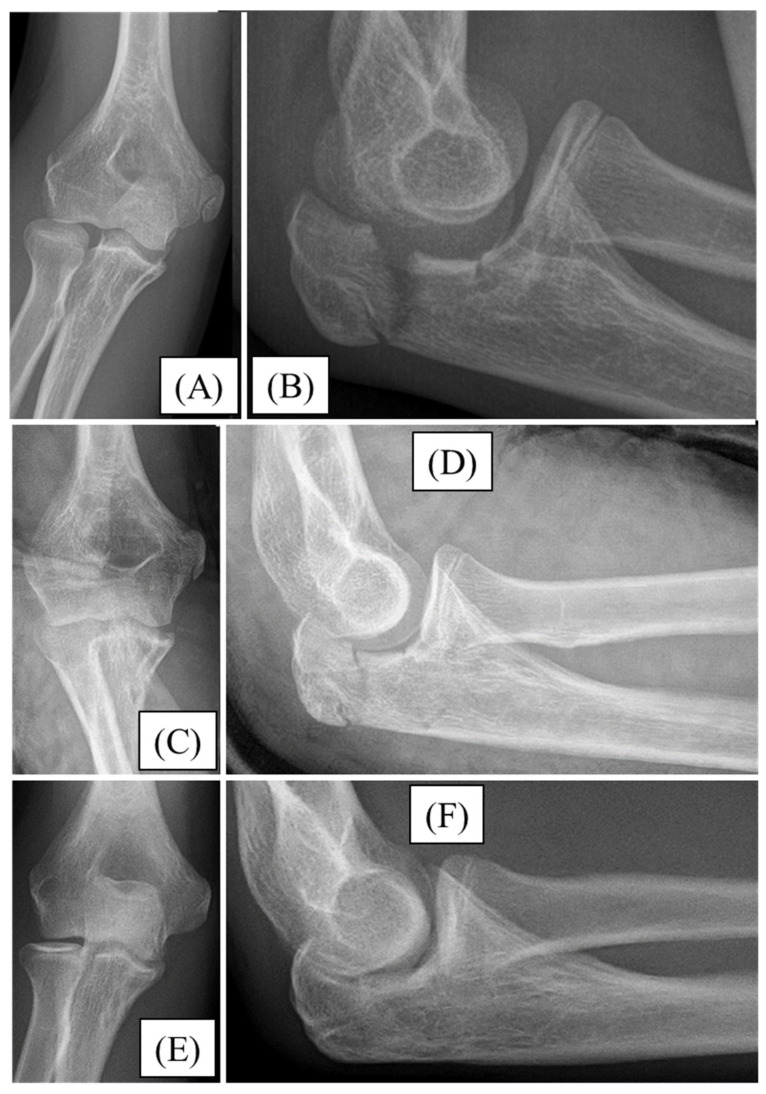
Initial X-rays of the right olecranon fracture (AP (A), lateral (B)), and the postoperative radiographs show adequate articular alignment (AP (C), lateral (D)). One year later the X-ray images (AP (E), lateral (F)) depict good alignment and acceptable congruency.
